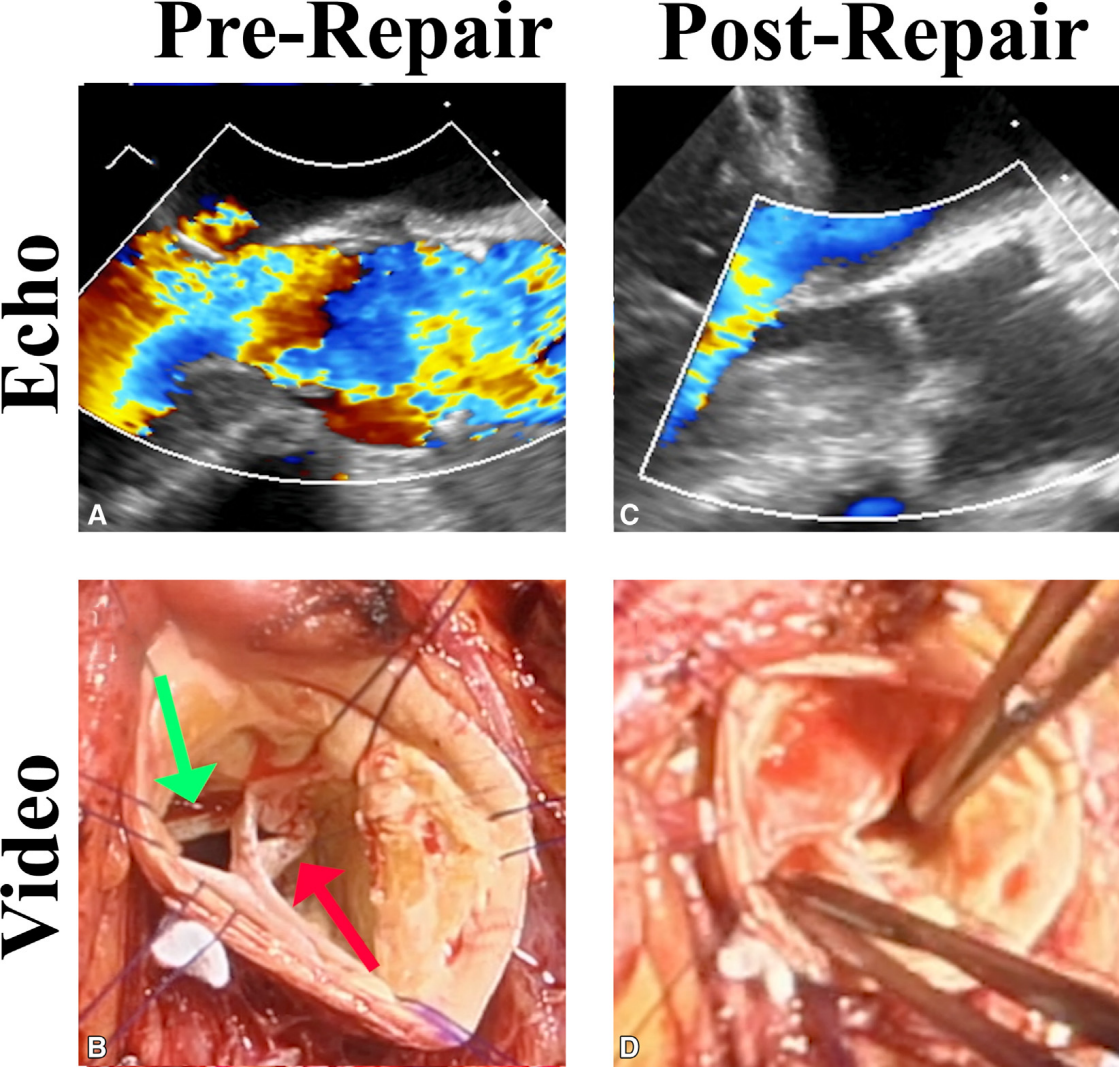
A, Prerepair echocardiogram showing severe AI. B, Prerepair pathology with a perforation in the noncoronary leaflet (*red arrow*) and an absent right coronary leaflet (*green arrow*). C, Postrepair echocardiogram showing no AI. *D*, Good matching and coaptation of the neoright coronary leaflet of aortic wall with the other native leaflets. *AI*, Aortic insufficiency.
